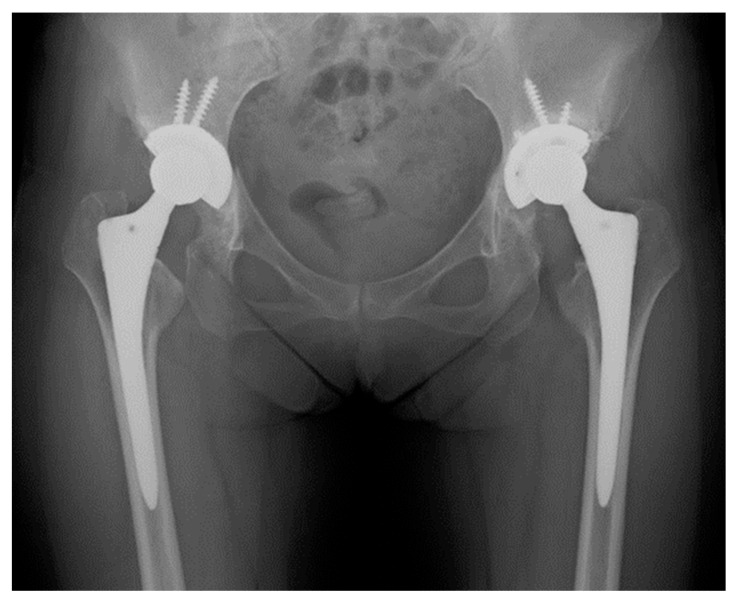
Plain X-ray of a 76-year-old woman, 15 years after THA; we evaluated this case as grade IA (no loosening) on the right side and as grade IB (radiolucent line was observed only in the outer one-third area) using the DeLee and Charnley classification.
